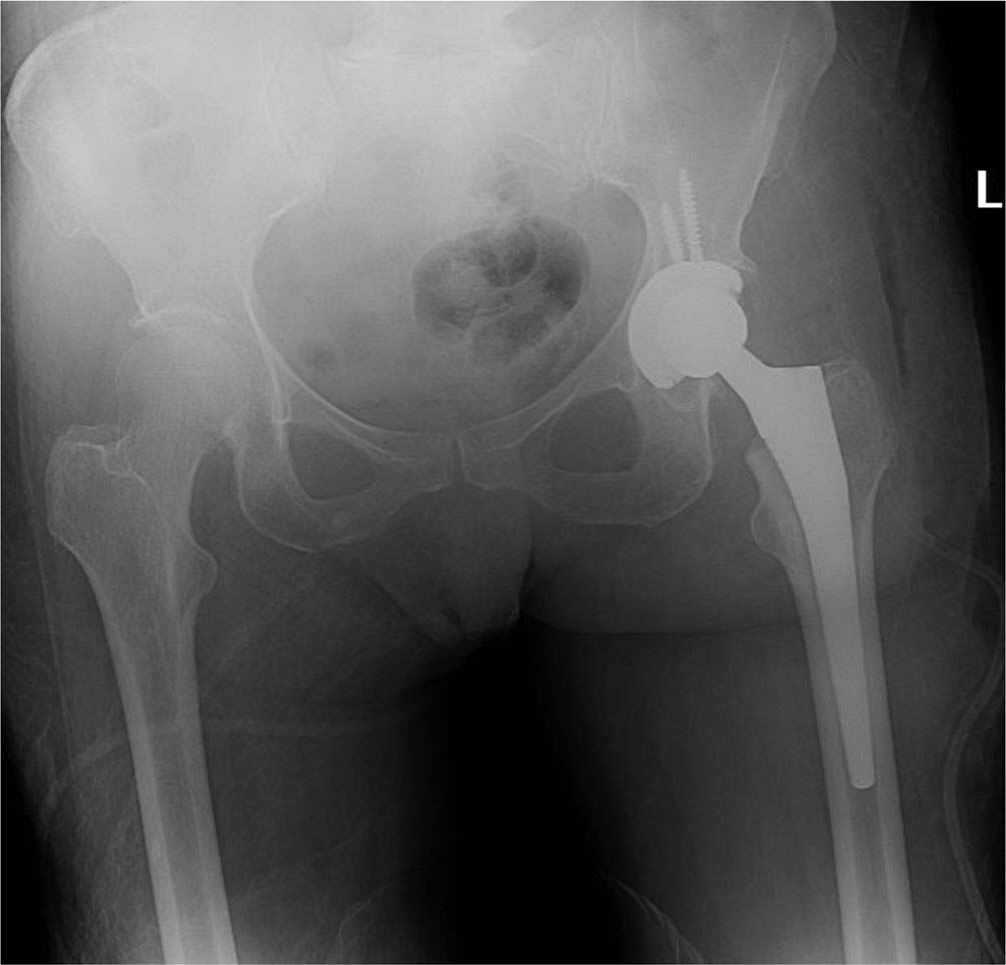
X-ray of the pelvis showing metallic hardware in good position.
